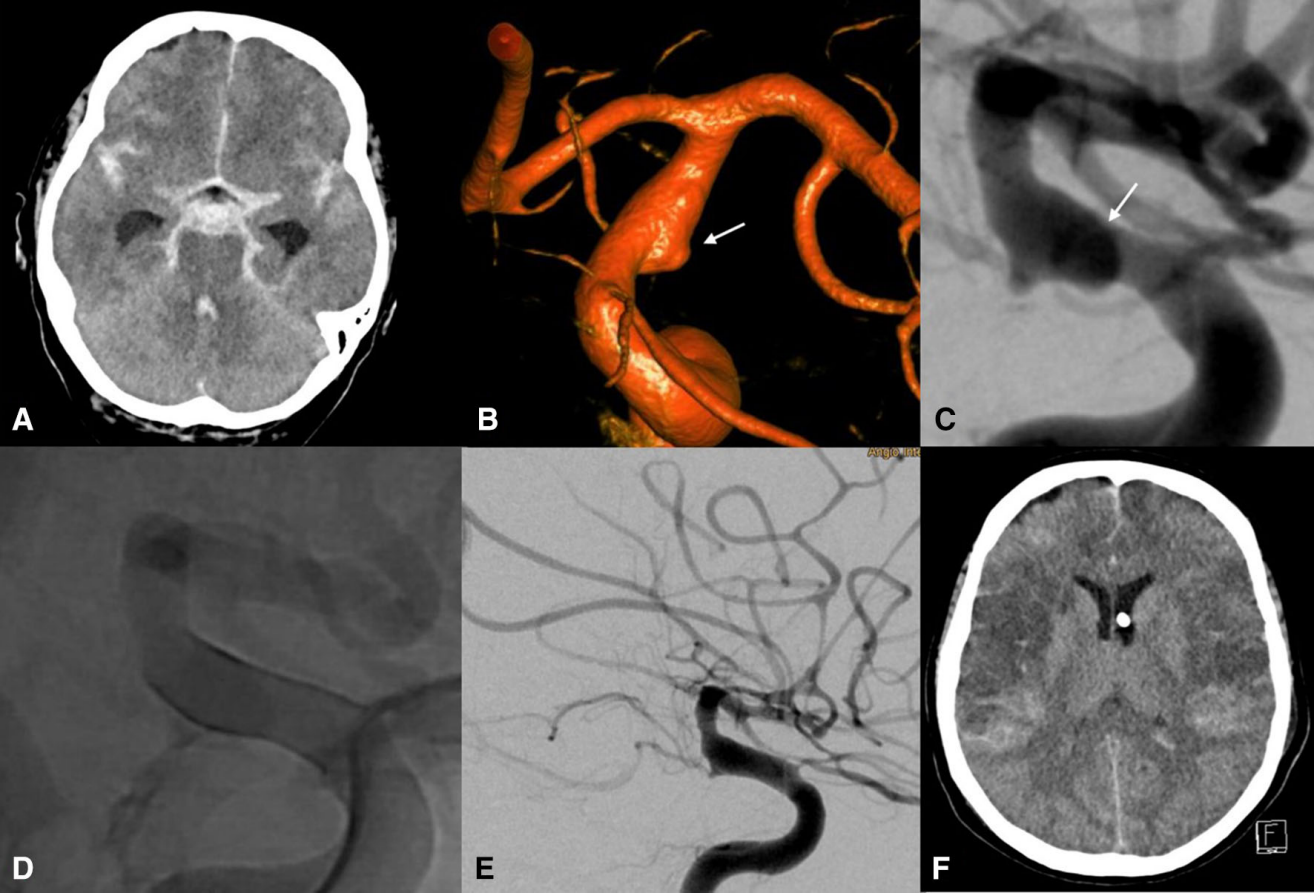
A 49-year-old female presented with sudden onset of severe headache and subsequent obtundation (Patient #8). Cranial CT scan (A) showed diffuse subarachnoid hemorrhage (Fisher grade 4) and hydrocephalus. A diagnostic cerebral angiography (B, 3D-Volumen Rendering Technique; C, lateral view) demonstrated a fusiform, most likely dissecting left ICA paraclinoid aneurysm with a non-discernible neck (B, C, arrow). The aneurysm was treated with a single p48MW HPC (3 mm × 18 mm) after premedication with 30 mg prasugrel PO 3 h before the procedure (D). Multiplate and VerifyNow test confirmed sufficient platelet inhibition before and after treatment. Angiographic follow-up performed on day 2 after treatment showed the patency of the FDS and intracranial massive cerebral vasospasm (E). Refractory cerebral vasospasm resulted in large bilateral MCA and ACA infarction (F) and eventually, death
